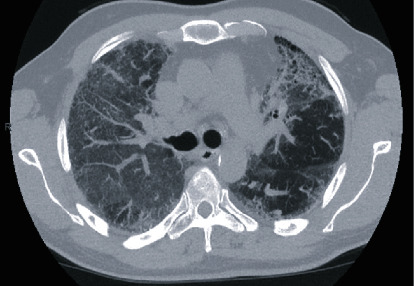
CT chest pretransplant (28/04/2015) demonstrating a UIP pattern of IPF.
